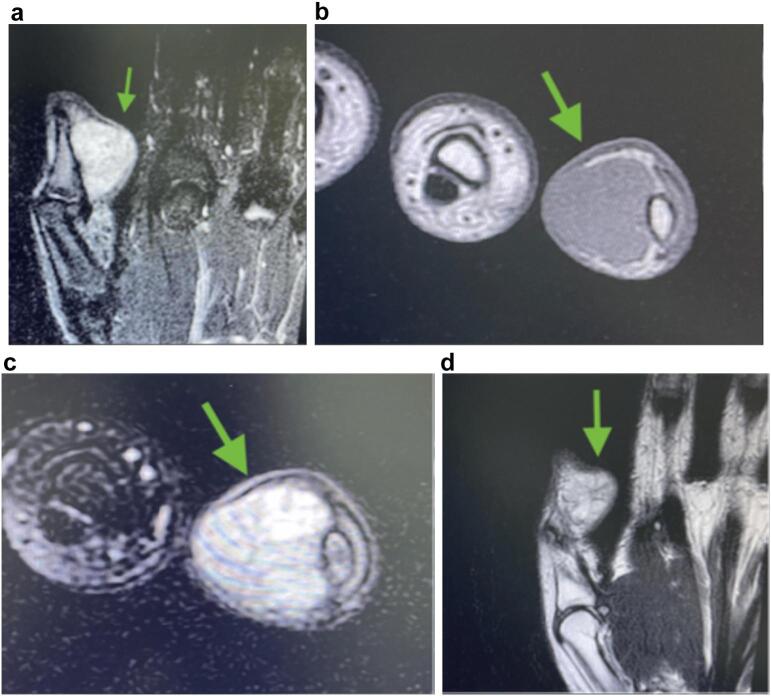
Magnetic Resonance Imaging of the left thumb [coronal view of STIR sequence (A), axial view of Contrast-enhanced T1-weighted sequence (B), axial view of STIR sequence (C) and T2-weighted sequence (D)] showing hyperintense areas on palmar side of the distal thumb.
